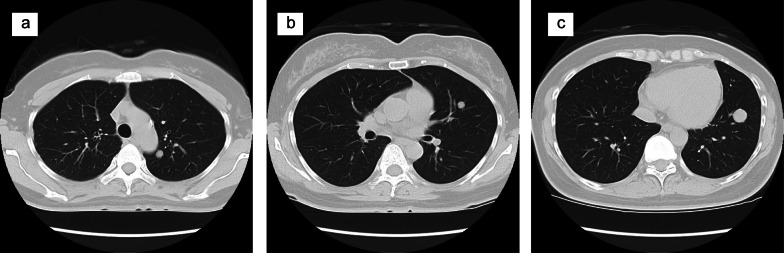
CT imaging of metastases, 10 years after first resection. a CT shows 8-mm nodular shadow on the upper left lobe; b another 8-mm nodular shadow on the upper left lobe; c 15-mm nodular shadow on the lower left lobe
